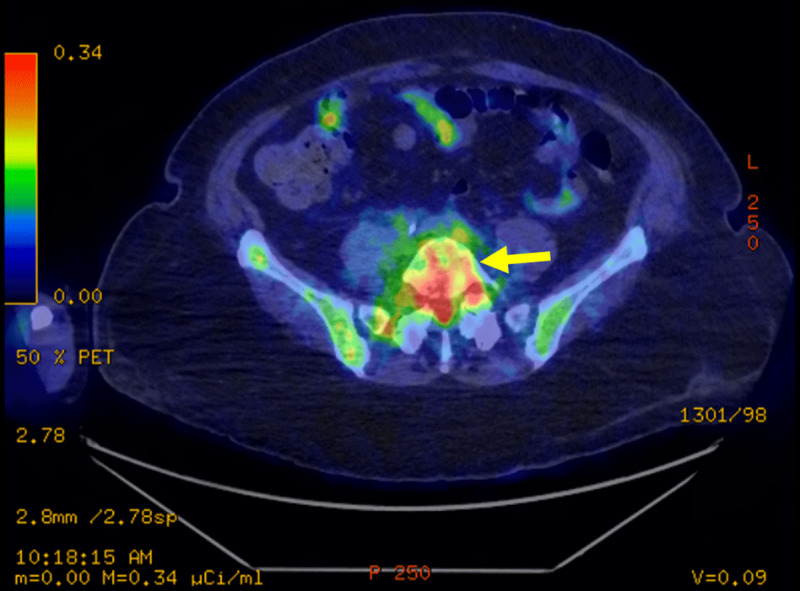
Transverse FDG PET-CT: PET-CT showing hyperfixation of L5-S1 disk.
